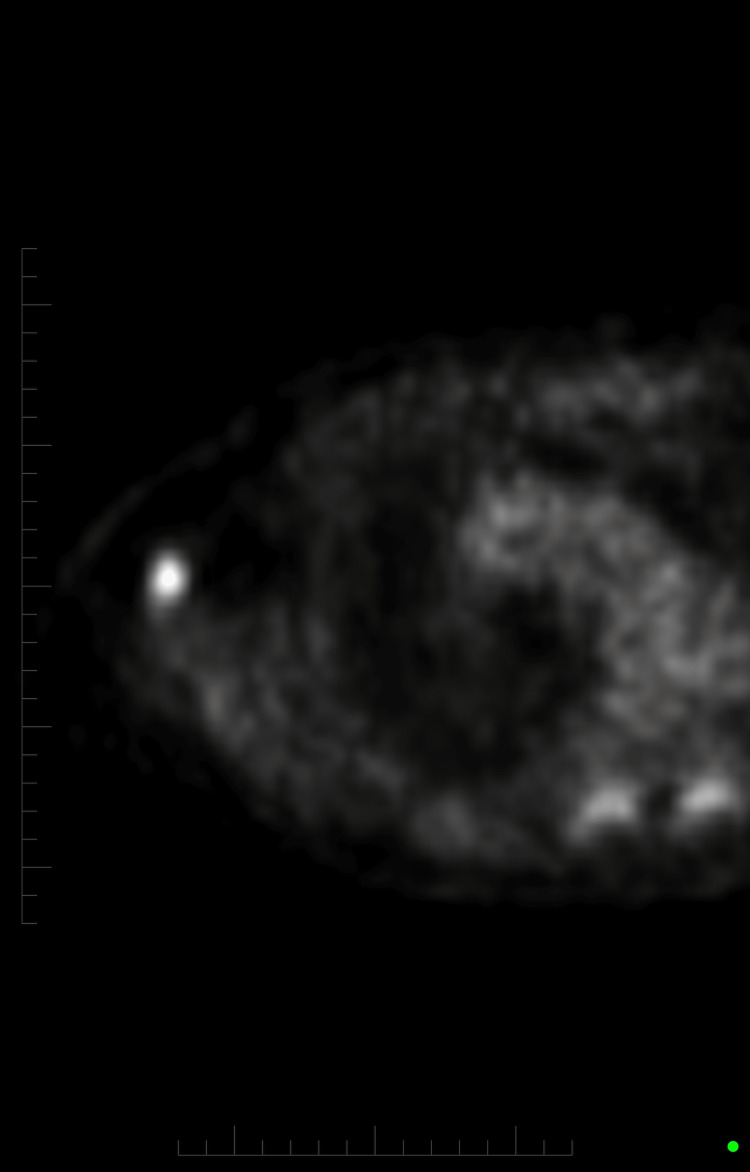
PET-CT showing hypermetabolic process. The radioactive uptake is suggestive of this process confirming metastasis. PET-CT: positron emission tomography-computed tomography
